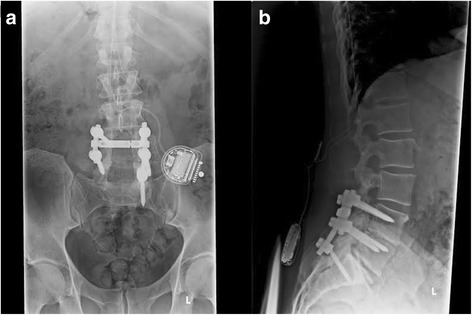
Radiographs of a lumbar spine status post lumbar decompression, fusion, and implantation of spinal cord stimulator. Visualized is a series of two radiographs, including an anterioposterior view (a), and a lateral view (b), of a post-surgical lumbar spine with pedicle screw and rod fixation at L4-S1 levels, and spinal cord stimulator lead wires entering the spinal column at L1-2 with a pulse generator over the left iliac crest
